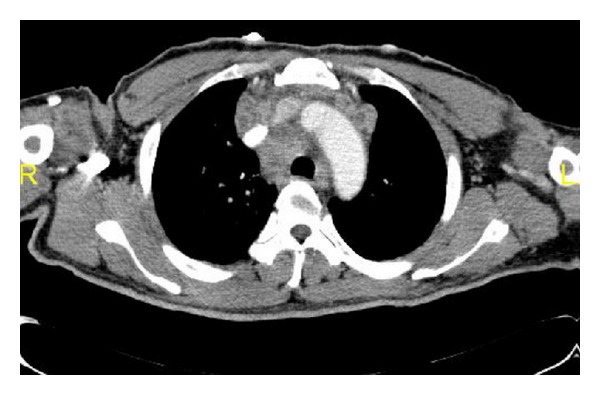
Computed tomography of chest showing mediastinal adenopathy with peripheral enhancement and central necrotic area.
